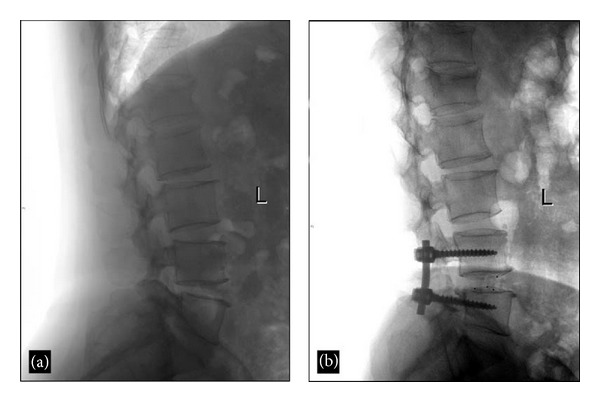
Lateral radiographs of the lumbar spine in a 55-year-old male with degenerative spondylolisthesis. (a) Intervertebral space at the L4-L5 level was narrowed, as shown on preoperative radiography. (b) The intervertebral space was restored after TLIF.
